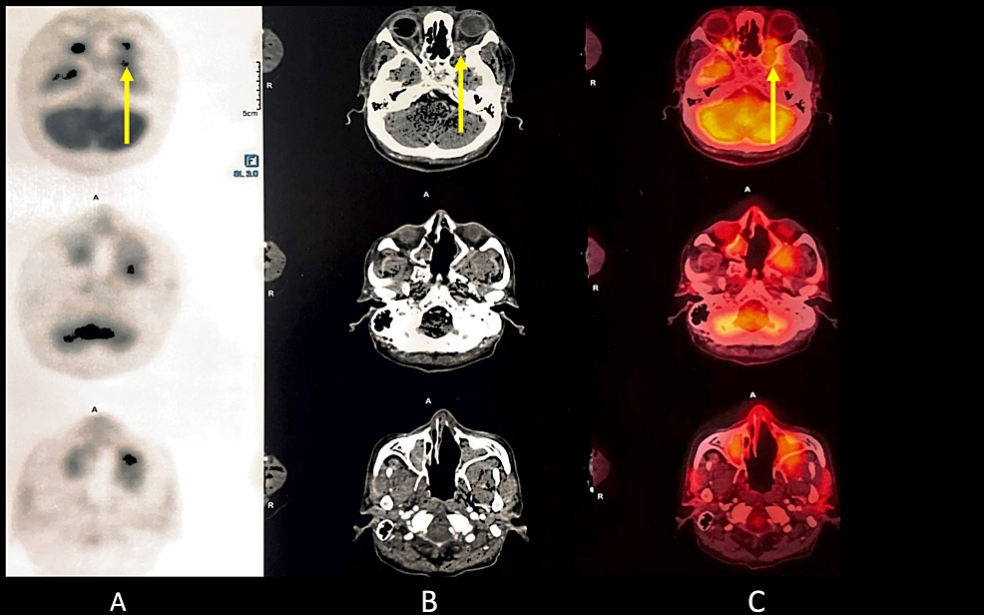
Positron emission tomography-computed tomography scan A. Positron emission tomography-computed tomography scan of the head reveals an ill-defined minimally enhancing mass lesion in the masticator space on the left side, showing low-grade diffuse fluorodeoxyglucose uptake with standardized uptake value max of 6.1 with obliteration of the retroantral fat on the left side. There is a superior extension of the mass reaching up to the apex of the left orbit (yellow arrow). B. Contrast-enhanced computed tomography scan of the head reveals an ill-defined minimally enhancing mass lesion in the masticator space on the left side, showing low-grade diffuse fluorodeoxyglucose uptake with standardized uptake value max of 6.1 with obliteration of the retroantral fat on the left side. There is a superior extension of the mass reaching up to the apex of the left orbit (yellow arrow). C. Positron emission tomography-computed tomography fusion scan of the head reveals an ill-defined minimally enhancing mass lesion in the masticator space on the left side, showing low-grade diffuse fluorodeoxyglucose uptake with standardized uptake value max of 6.1 with obliteration of the retroantral fat on the left side. There is a superior extension of the mass reaching up to the apex of the left orbit (yellow arrow).
